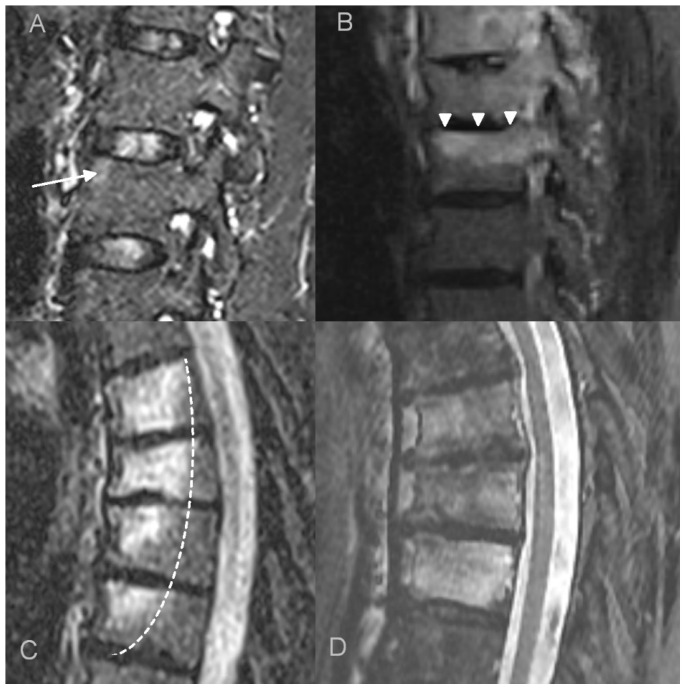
Bone marrow edema (BME) patterns appreciated on T2 FS/STIR MRI images. (A). corner inflammatory lesions (arrow); (B). propagating (arrowheads); (C). semicircular/curvilinear (dotted line); (D). diffuse—patchy (in the upper two vertebrae) and solid (in the lowest vertebra).
